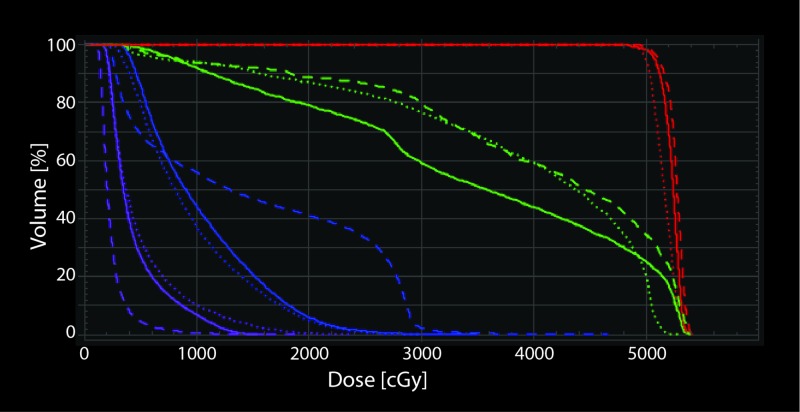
Dose-volume histogram for the three treatment plans used to treat this patient. This assumes all 30 fractions were delivered with each plan. First plan with VMAT (solid line), second plan with VMAT (dotted line), and third plan with FFB (dashed line) are shown with prescriptions set to 52.5 Gy in 30 fractions for comparison. PTV (red), duodenum (green), left kidney (blue) and right kidney (purple) are shown. FFB plan has higher doses to the organs at risk, except for the right kidney. PTV: Planning target volume; CBCT: Cone-beam computed tomography; FFB: Four-field box; VMAT: Volumetric modulated arc therapy.
